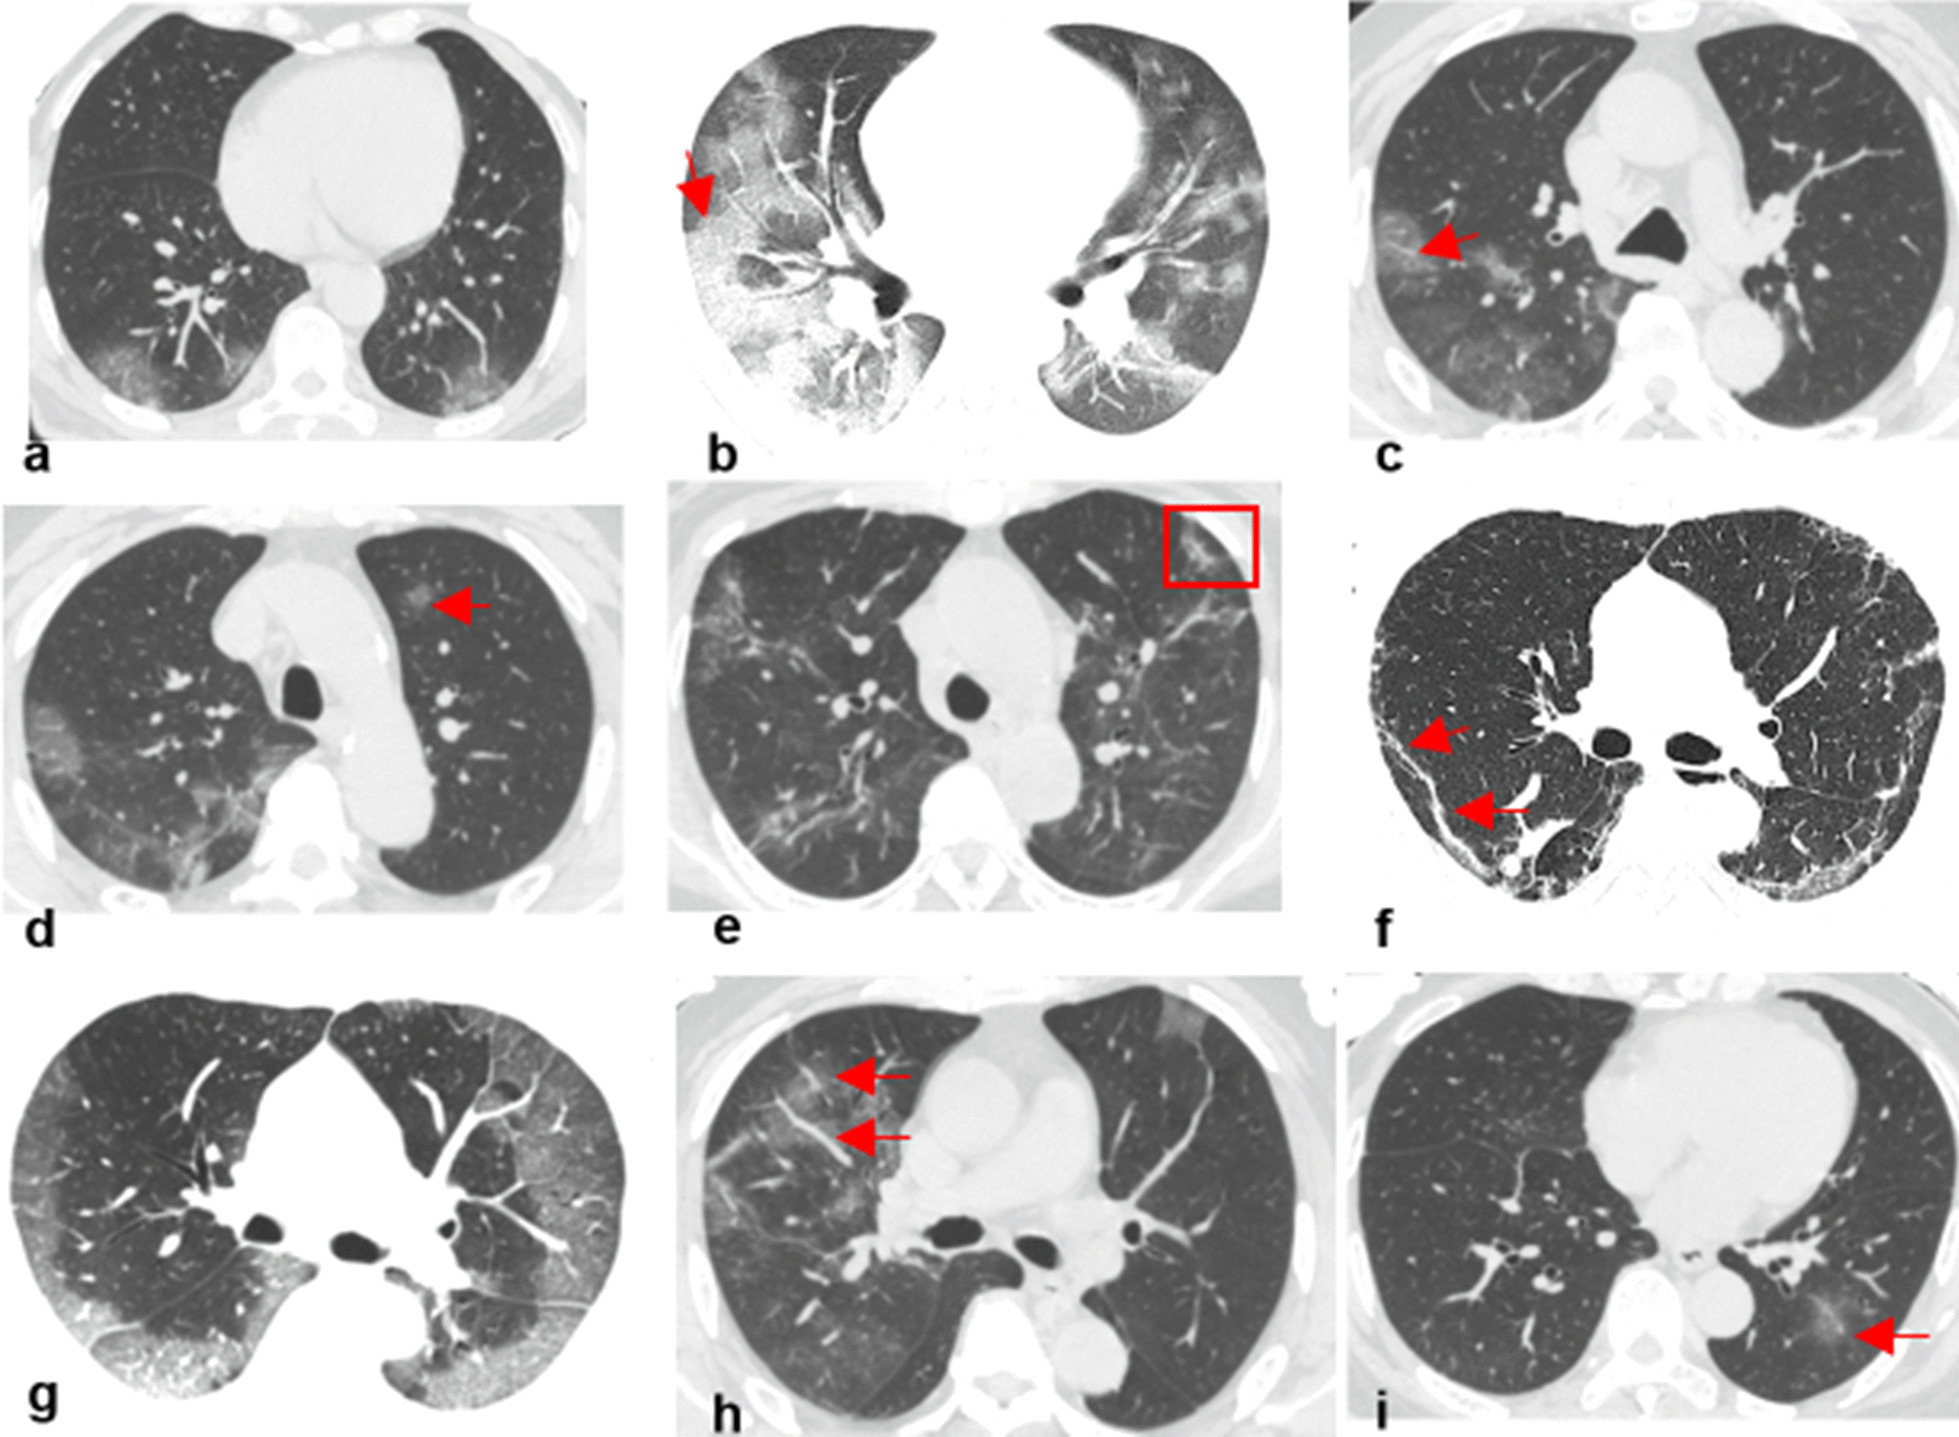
Typical radiological characteristics of CT manifestations. a–i demonstrated the typical radiological characteristics of ground grass opacity, crazy paving pattern, halo sign, reversed halo sign, vascular perforating in the lesion, subpleural line, subpleural distribution, broncho vascular bundle distribution, and pulmonary band distribution, respectively
